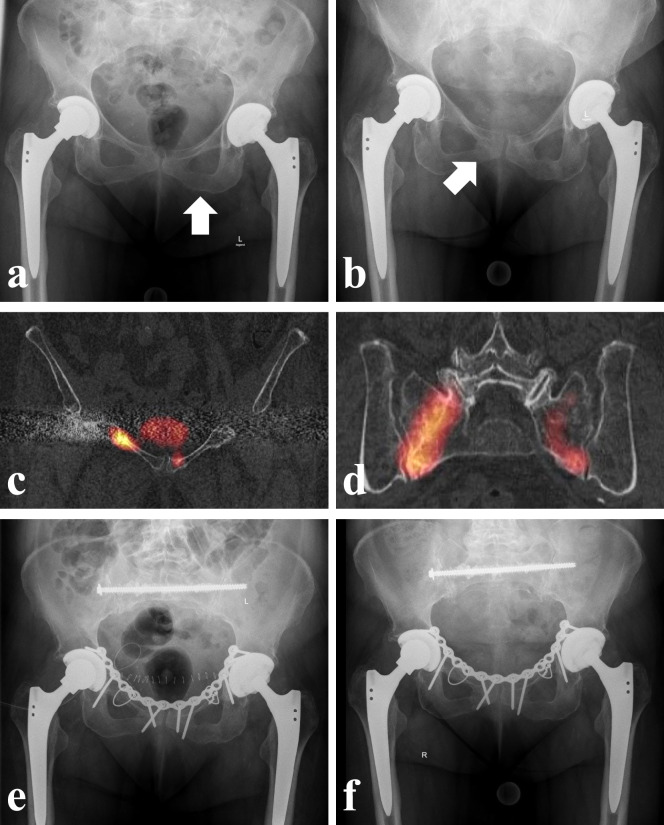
77-year-old female after lateral fall showed in ap pelvis (a) a non-displaced pubic ramus fracture on the left side. Conservative treatment with 6 weeks partial weight bearing on the left side was initiated. 6 months later, the patient suffered a new non-displaced pubic ramus fracture on the right side with associated bilateral sacral insufficiency fractures as sign of the crescendo effect of osteoporotic pelvic fractures. Ap pelvis (b) and coronal reconstructions of SPECT-computed tomography of the anterior pelvic ring (c) and the posterior pelvic ring (d). The anterior pelvis was fixed with a reconstruction plate with additional bilateral cerclages around the pubic rami to increase the stability and prevent loosening of the screws. The posterior lesions were stabilized with a cement-augmented transsacral screw (ap pelvis postoperatively (e) and 1 year postoperatively (f)
